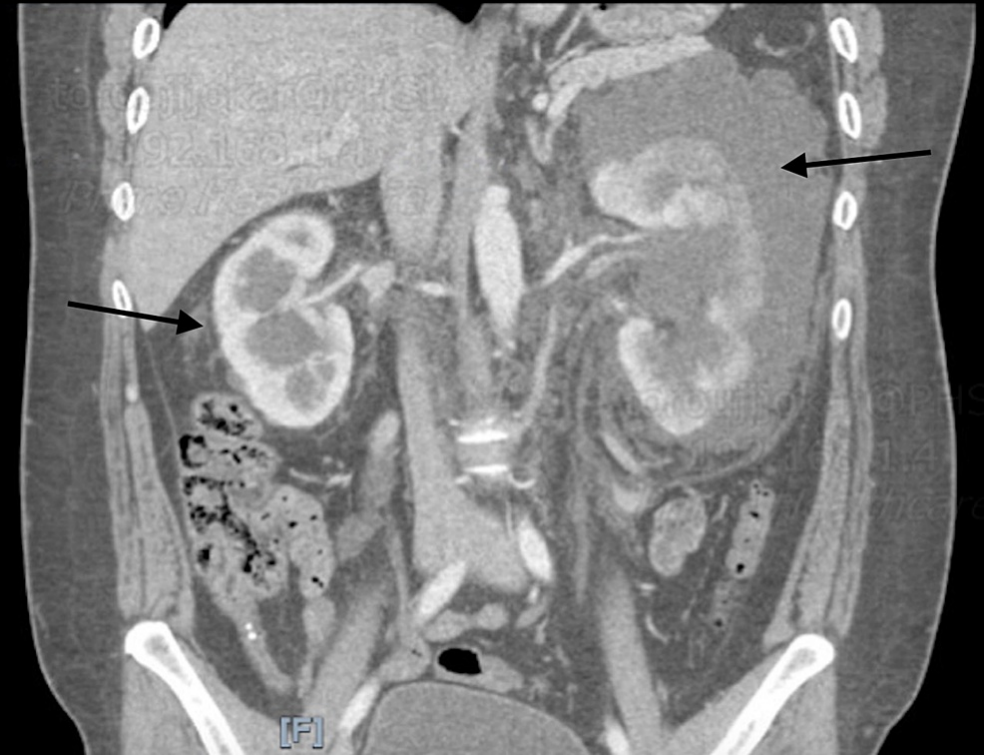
CT findings of perirenal lymphangiectasia: arrows pointing to bilateral perinephric lymphangiectasia with more pronounced changes on the left
